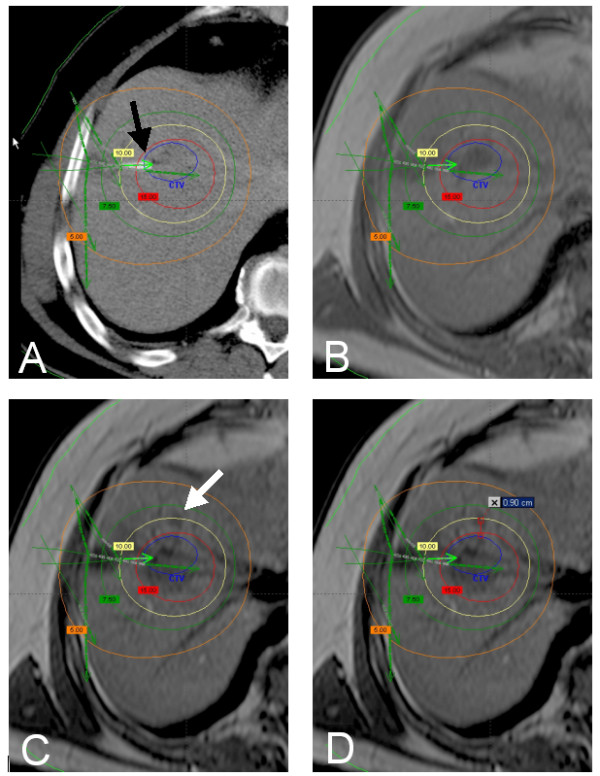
A: planning CT with overlayed dosimetry (BrachyVision^®^) showing a colorectal liver metastasis in segment 8. One catheter tip is displayed directly (black arrow), more catheters in other levels of the liver are indicated by green arrows. Verification of correct definition of the CTV was performed by image fusion of the planning CT with a MR scan (T1 GRE without contrast media) obtained 3 days prior to treatment (B). Local recurrence (white arrow) 6 months after treatment (MR, T1 GRE without contrast media, C). The distance of the 3D isocenter of the local recurrence from the initial tumor margin is 9 mm. Thus, the local tumor recurrence meets the criteria for micrometastasis growth (D). The dose initially applied in the center of the micrometastasis was 10.9 Gy.
